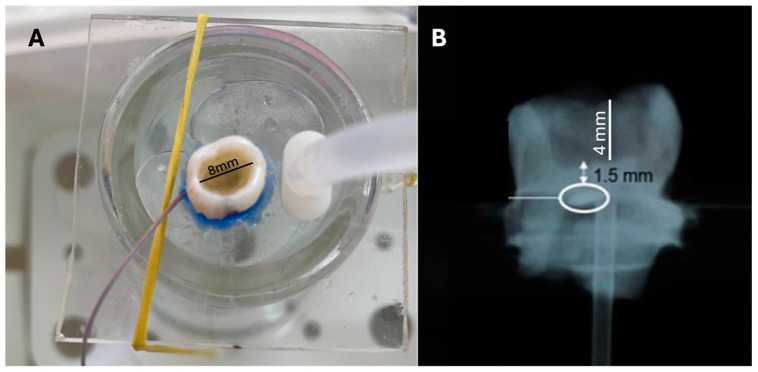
(A): Tooth with the divergent Class I cavity positioned in the Kitasato bottle. The cavity dimensions were 8 mm wide and 7 mm long. (B): Radiograph of the position of the thermocouple. The remaining dentin thickness was 1.5 mm, and the cavity was 4 mm deep. The white circle represents the position of the thermocouple inside the pulp chamber.
